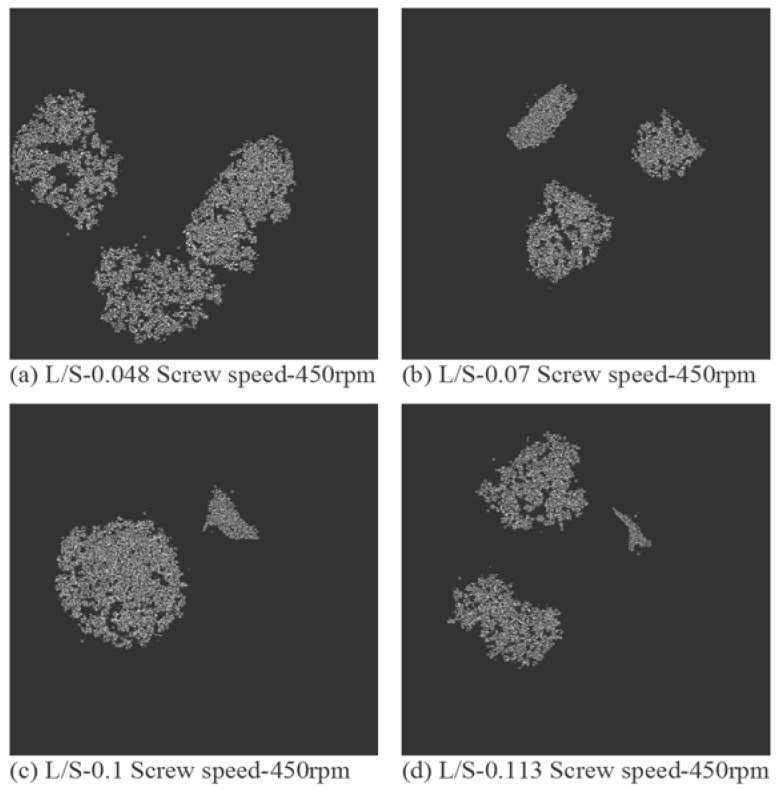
X-ray tomographic images of granules produced using Pearlitol 200SD at varying L/S (screw speed, 450 rpm).
